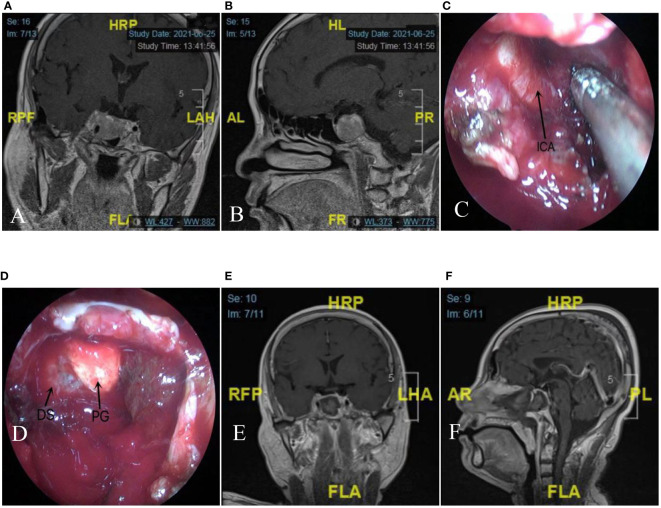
A case of giant pituitary adenoma (Knosp Grade 4) with right cavernous sinus invasion and encasement of ICA. The binasal speculum will be taken out to perform the deep lateral regions during the later stage. (A, B) T1-weighted MRI with gadolinium enhancement [(A): coronal section, (B) sagittal section]. (C) The patient underwent eendoscopic endonasal surgery using the binasal speculum, a full exposure of ICA. (D) After gross total removal of the tumor, the normal anatomical structures, including the diaphragma sellae drop and the normal pituitary gland are visualized. (E,F) Postoperative T1-weighted MRI with gadolinium enhancement confirmed a minor residual tumor in the right cavernous sinus. [(E) coronal section, (F) sagittal section]. ICA, internal carotid artery; DS, diaphragma sellae; PG, pituitary gland(black arrow).
